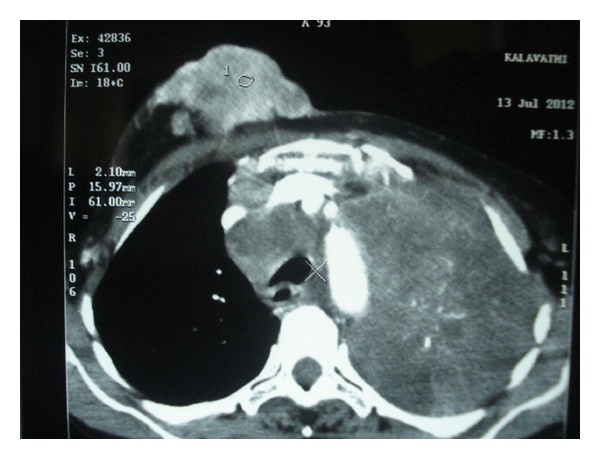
Contrast-enhanced computed tomography (CECT) chest showing left upper lobe lung mass with collapse and left mild pleural effusion. Irregular mass seen in the right breast with loss of fat planes between it and the chest wall.
